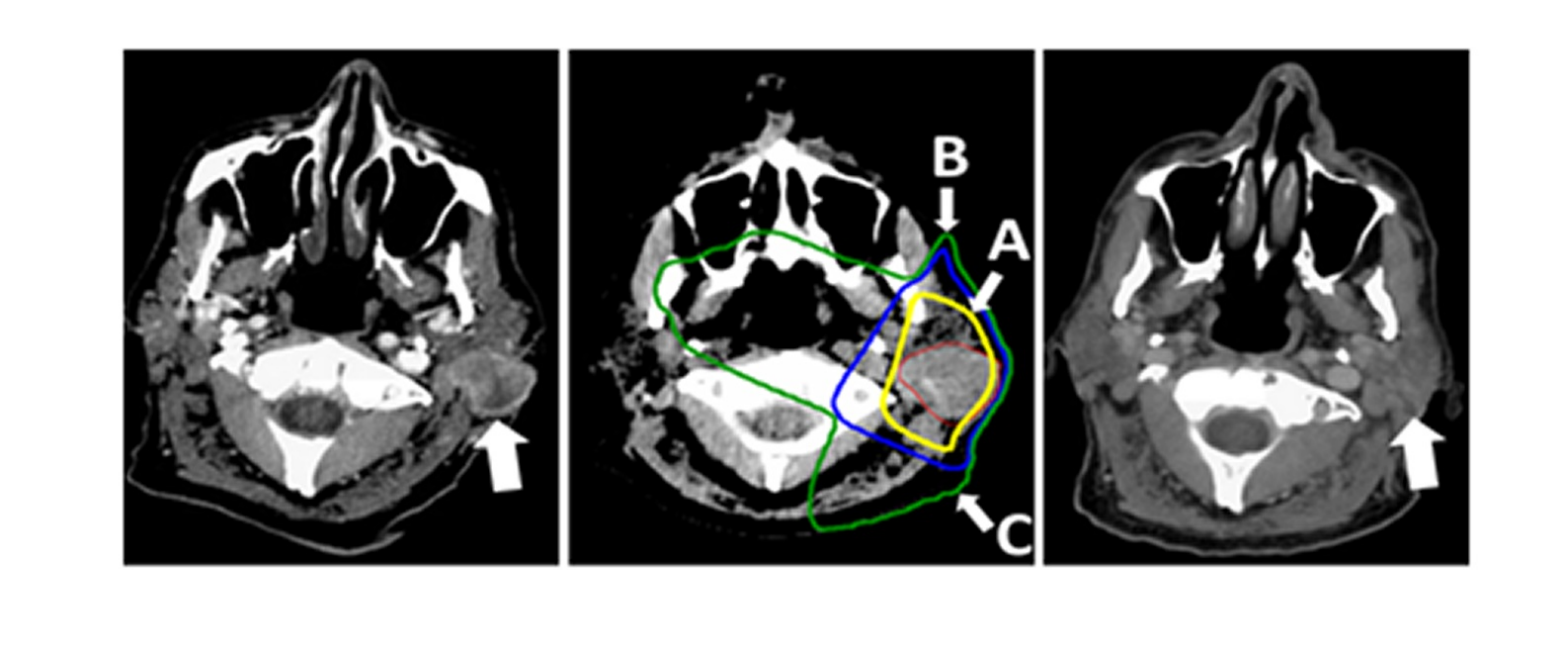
Axial Post-Contrast CT Images of the Primary Tumor Before and after Two Months Post-Treatment Axial post-contrast CT images of the primary tumor before (left, white arrow) and 2 months post treatment (right, white arrow). The involution in tumor size was so dramatic the radiology report was read as “status/post resection of the mass between the left sternocleidomastoid and parotid gland,” even though no surgery had been done. A representative image of the radiotherapy plan is also shown (center panel). 95% (A), 50% (B) and 30% (C) isodose lines are shown.
